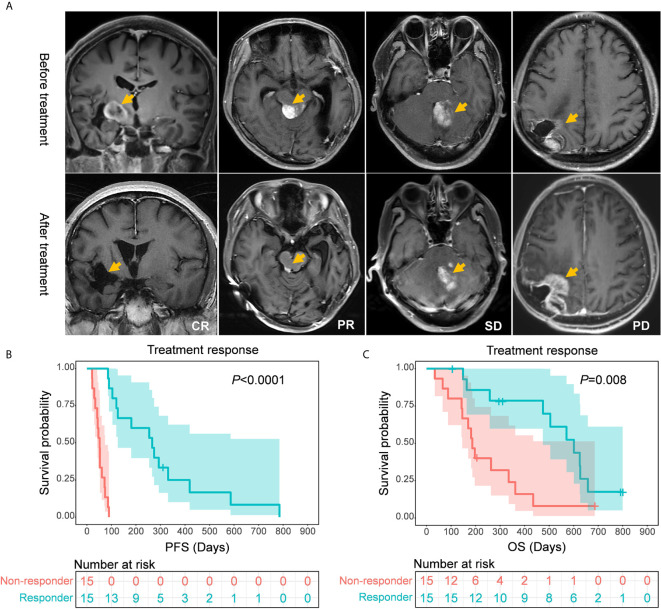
(A) Representative MR images of patients with different treatment responses. (B, C) Comparisons of survival rates between responders and non-responders. Responders showed a significantly longer progression-free survival (*P* < 0.0001) and overall survival (*P*=0.008) than non-responders.
